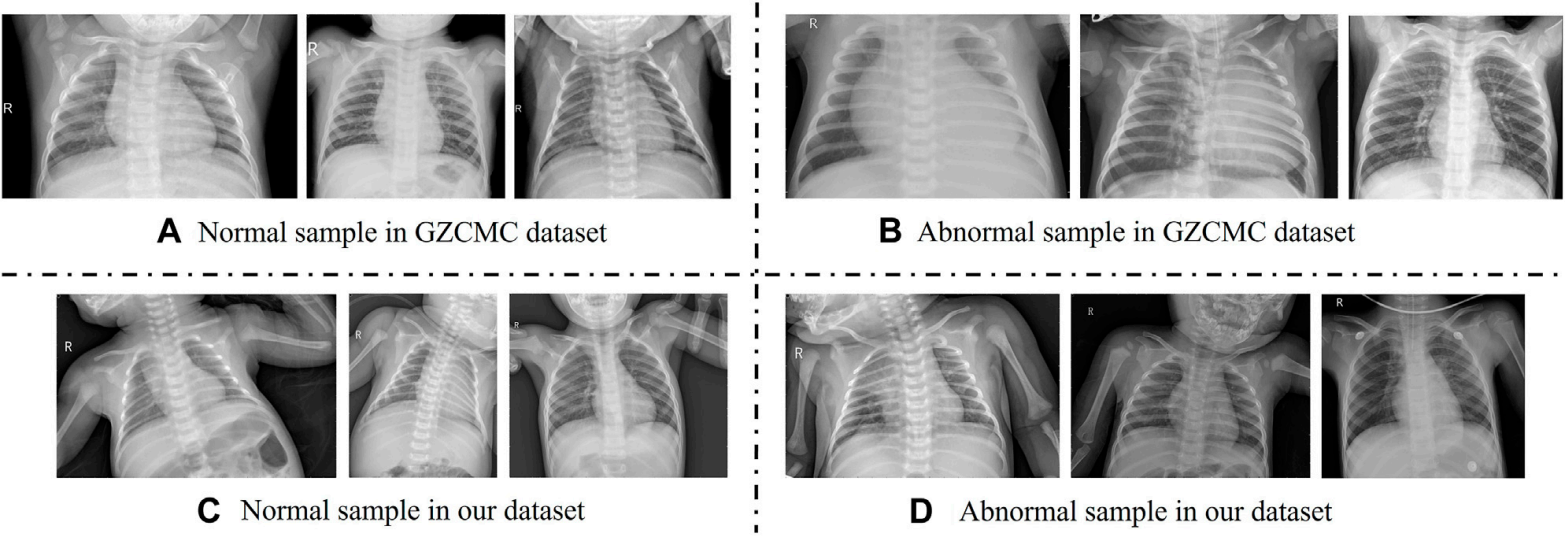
Comparison with adolescent and infant X-ray samples from the Guangzhou Women and Children’s Medical Center (GZCMC) Dataset. (A,B) Represent samples the GZCMC dataset. (C,D) Represent samples from our dataset.
